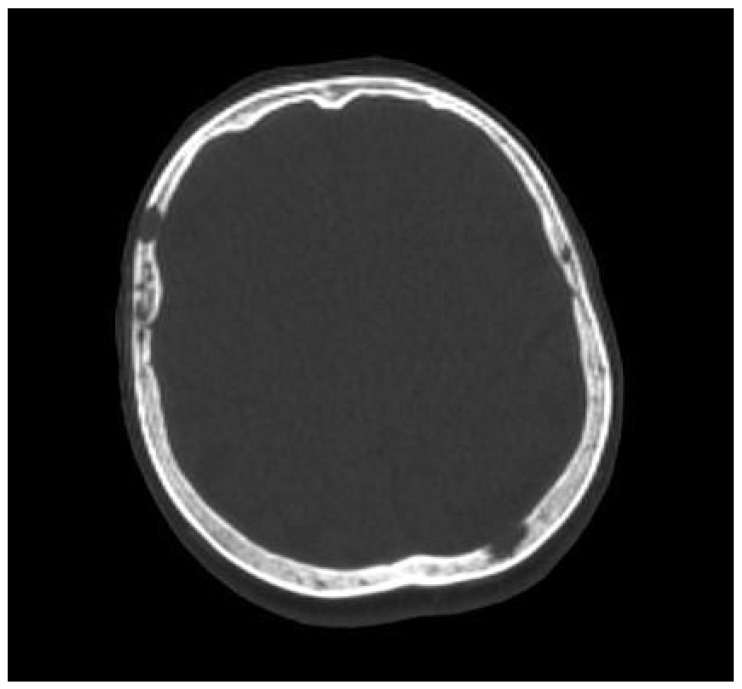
A 65-year old woman with multiple myeloma, presenting some lytic lesions in the bones of the skull on whole-body low-dose computed tomography. Source: Radiological Diagnostics Center, The National Institute of Medicine of the Ministry of Interior and Administration, Warsaw, Poland.
